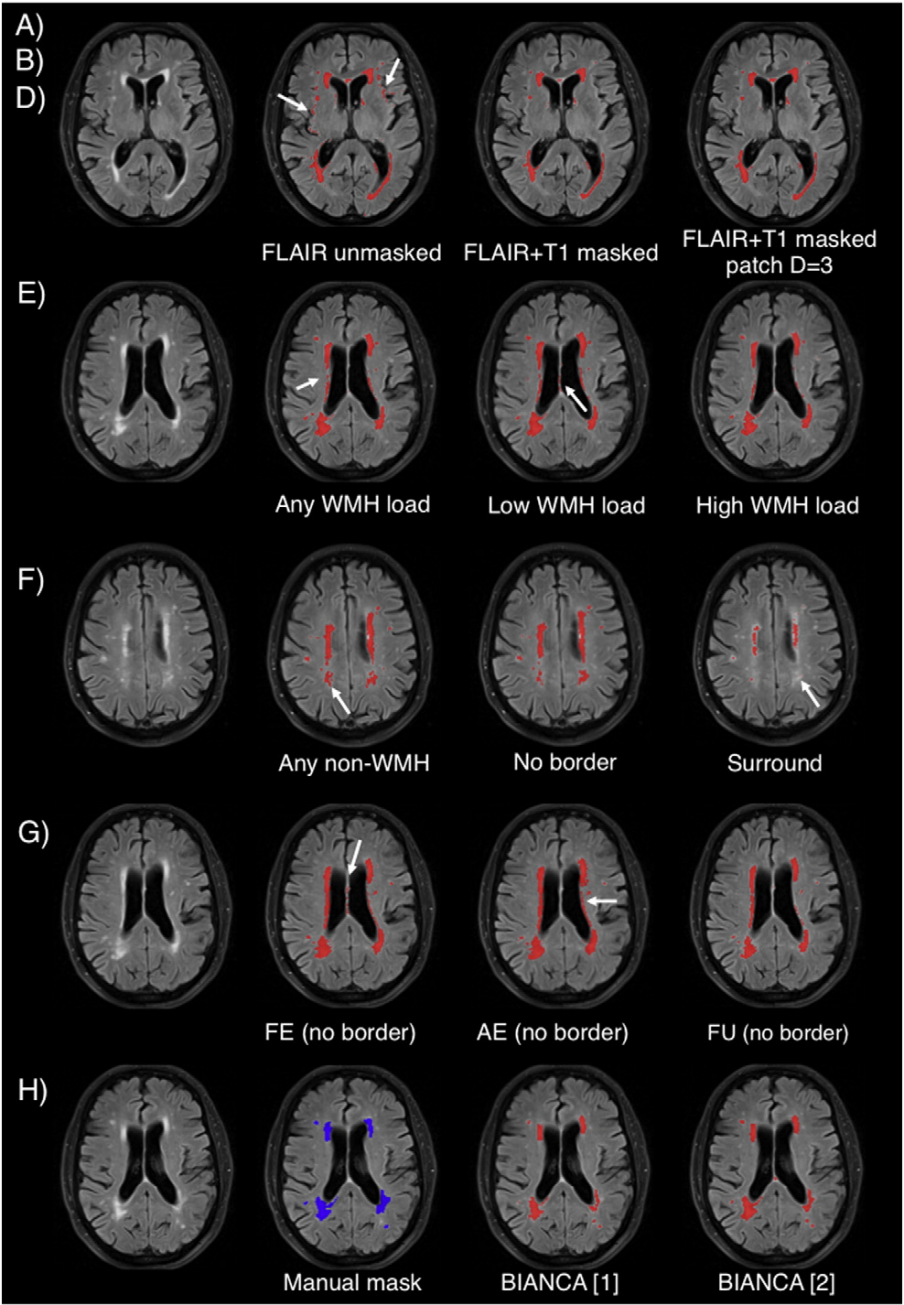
BIANCA optimisation. Examples of BIANCA output from some of the options tested (especially those not already evaluated in literature) on one subject from Dataset 1 (male, 76 years): A) and B) Multimodal MRI and masking; D) “patch” option; E) training subjects; F) localisation of training points; G) number of training points; H) combination of best options, shown next to the manual mask. The white arrows point at segmentation errors. Please refer to main text for details about the options.
